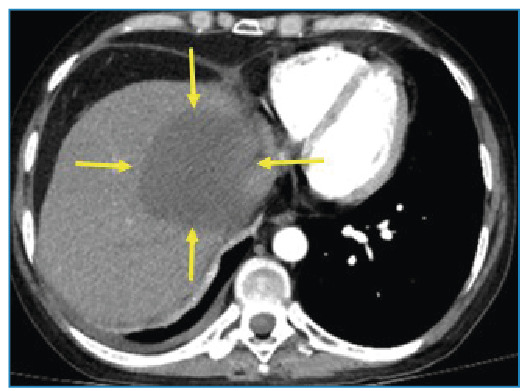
Axial plane CT showing large solitary hypodense lesion in liver parenchyma (yellow arrows).
